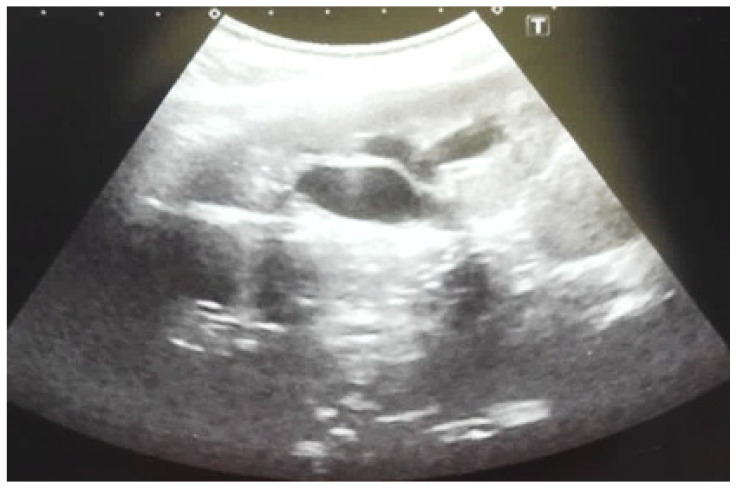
Ultrasound of the postnatal urinary system 10 days after birth. Hydronephrosis on the right.
